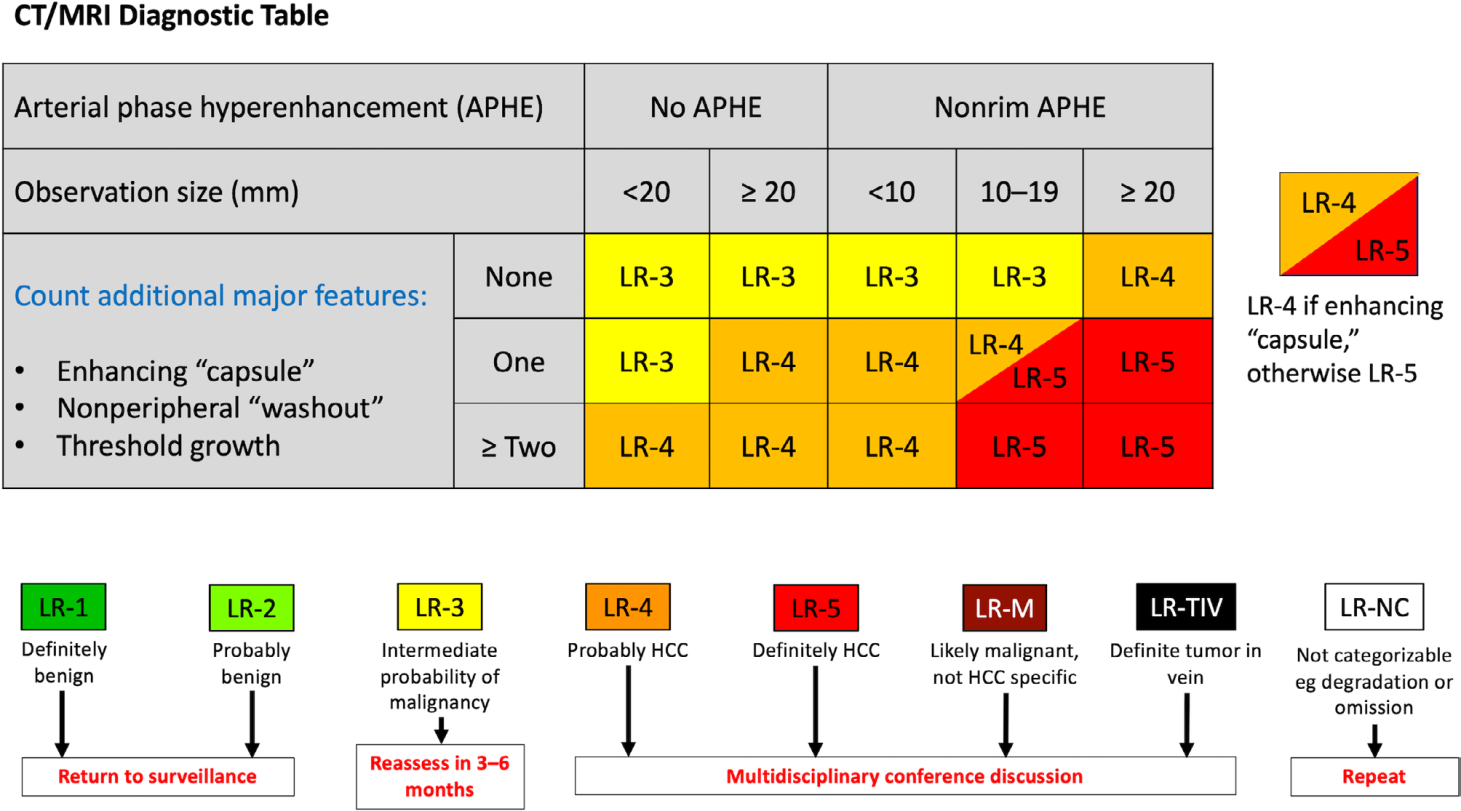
The American College of Radiology CT/MRI LI‐RADS version 2018 Diagnostic Table (Available at https://www.acr.org/Clinical‐Resources/Clinical‐Tools‐and‐Reference/Reporting‐and‐Data‐Systems/LI‐RADS). Below the table are descriptors of each category along with a summary of the corresponding management recommendation in red text.
